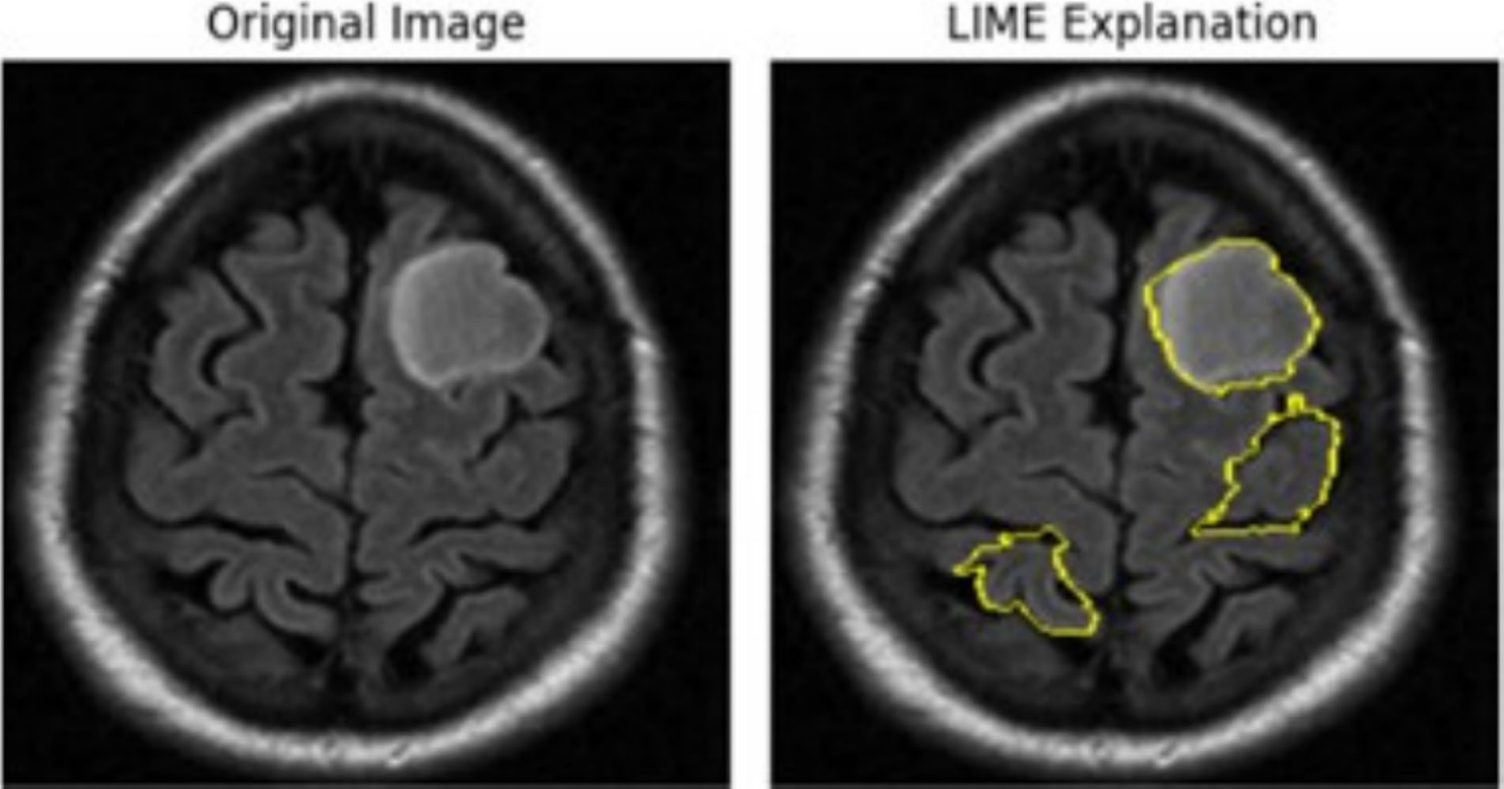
Glioma tumor MRI image highlighting key areas of the scan when passed through the 5-conv-3-dense-2-dropout model for classification.
